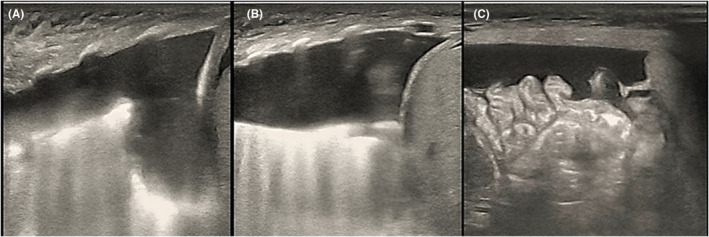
Ultrasound showed pleural effusion and seroperitoneum. (A) Left‐sided pleural effusion. (B) Right‐sided pleural effusion. (C) Seroperitoneum.
